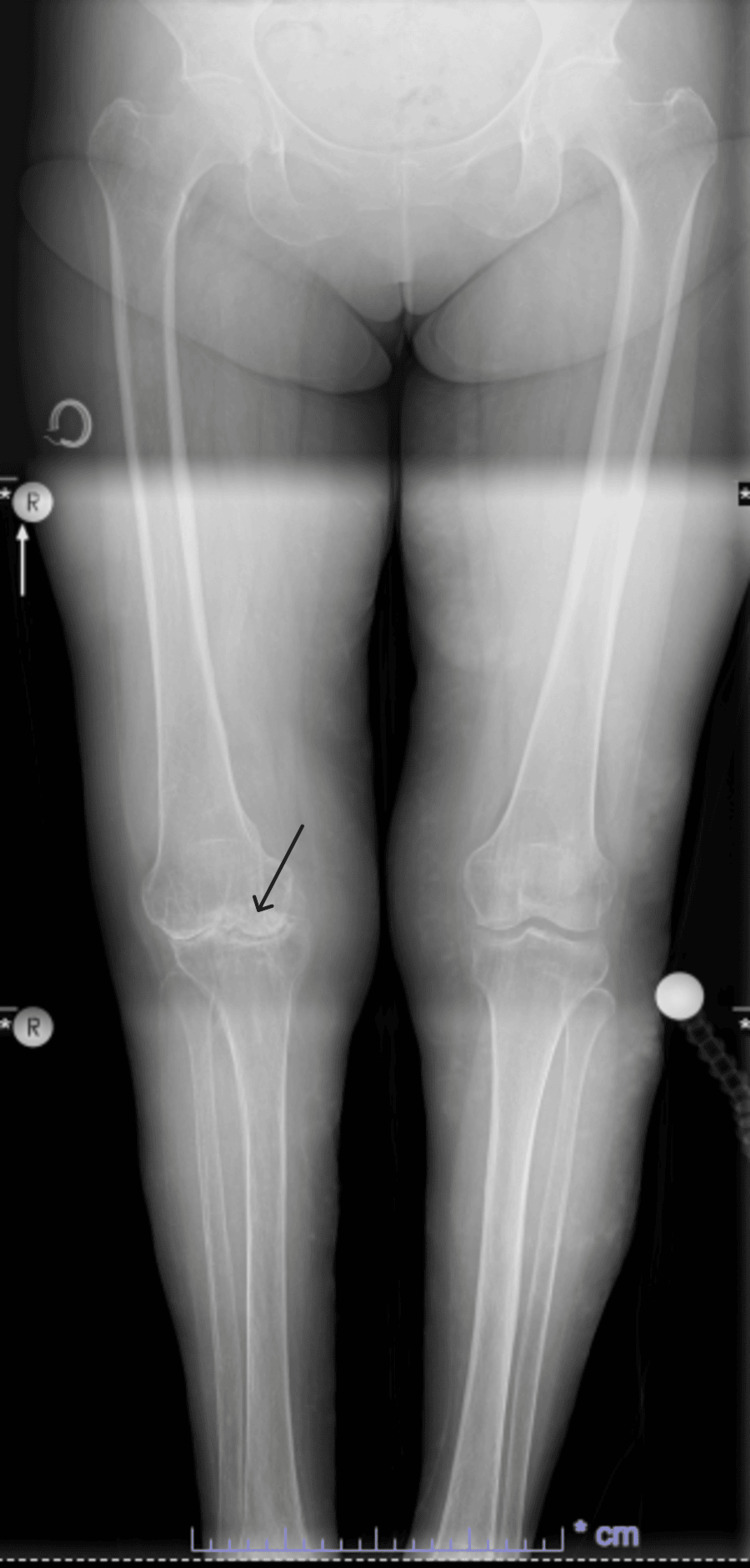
Plain radiography, AP, weight bearing view of bilateral knees. Presence of end stage arthritis of right knee.
